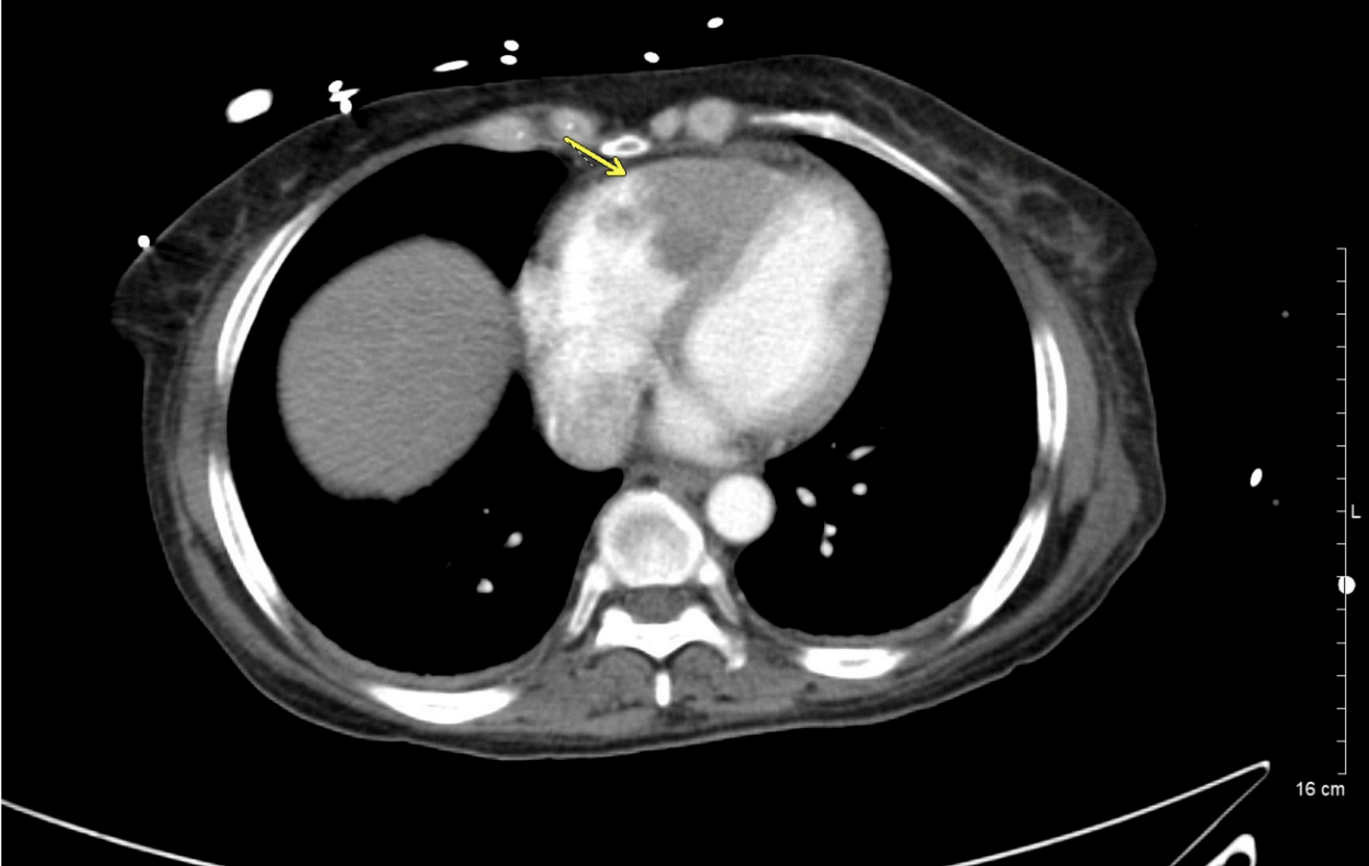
Repeat axial post-contrast CT of the chest showing persistent right ventricular thrombus (arrow), unchanged in size and morphology.
